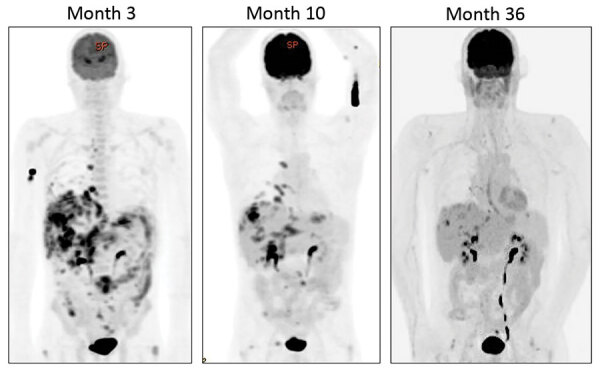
Serial ^18^fluorodeoxyglucose-positron emission tomography/computed tomography imaging quantification of disease burden for an HIV-positive person (case-patient 1) given treatment for disseminated *Mycobacterium abscessus* infection, London, UK. Images demonstrate marked reduction in fluorodeoxyglucose avidity over time.
